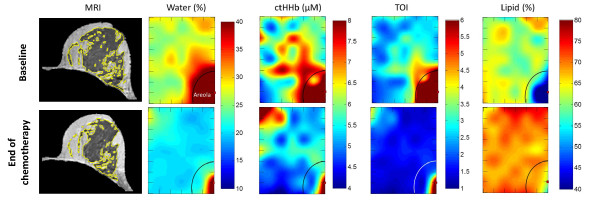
Corresponding magnetic resonance imaging (MRI) and diffuse optical
